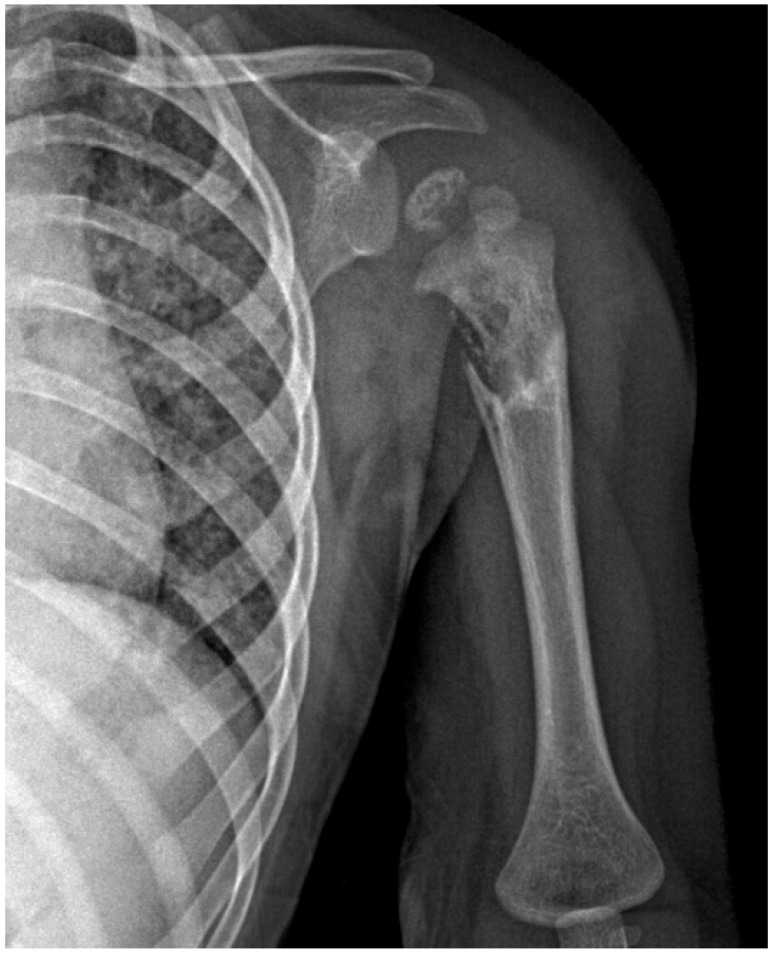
X-ray of the left shoulder: demineralization and irregular areas of osteolysis, with loss of uniform outline at the diaphyseal-metaphyseal and epiphyseal level, proximal of the left humerus.
